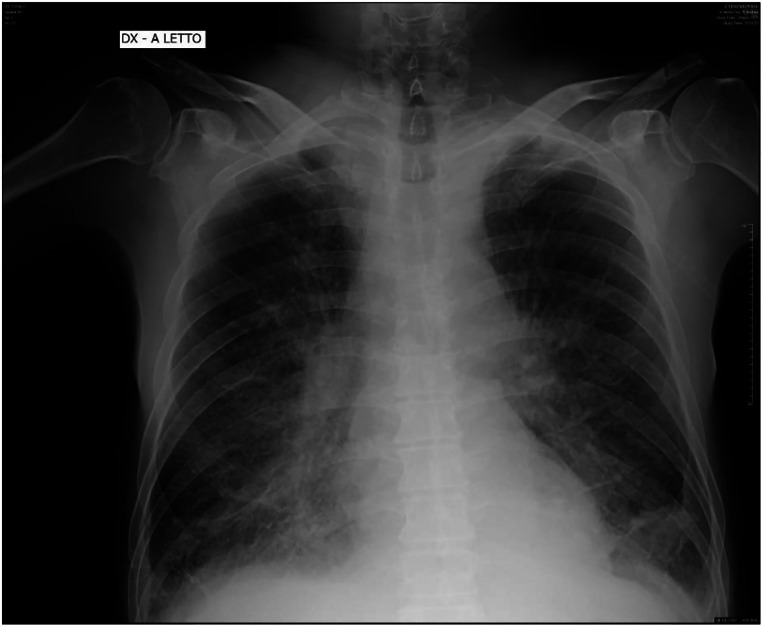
Emergency chest X-ray with severe interstitial edema
